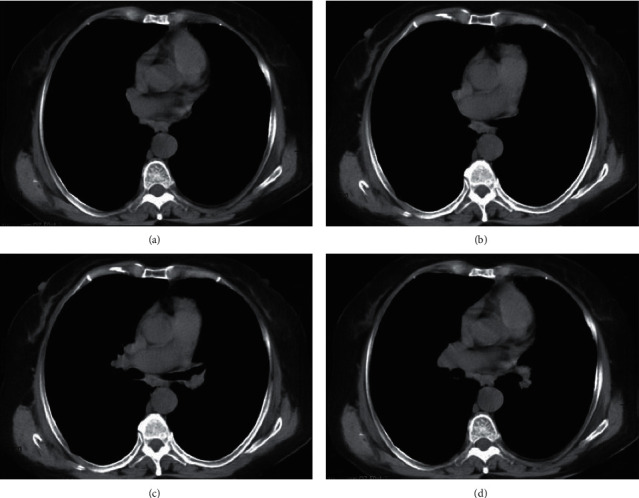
MRI images for PB patients. (a) MRI image of a 7-year-old male patient with chronic emphysema and pleural thickening and adhesion. (b) MRI image of a 10-year-old child with aortic dissection. (c) MRI image of an 11-year-old child with pleural calcification near the right upper mediastinum. (d) MRI image of a 10-year-old male child with double-cavity signs.
